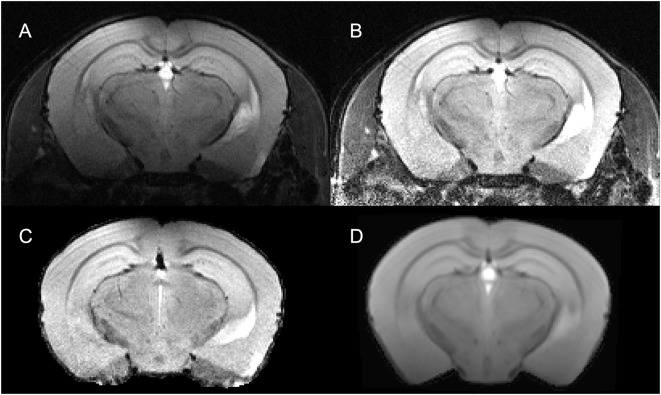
Visualization of step 2—pre-processing for a representative T2-weighted data set. The raw data set *I*^T^^2^(*x*) (A) underwent a re-orientation *f*_re_(*x*) and bias field correction using multiplicative intrinsic component optimization (MICO) *f*_bc_(*x*) to reduce inhomogeneities (B). The subsequent registration is done on a brain extracted volume $I_{\text{ex}}^{T2}(x)$
